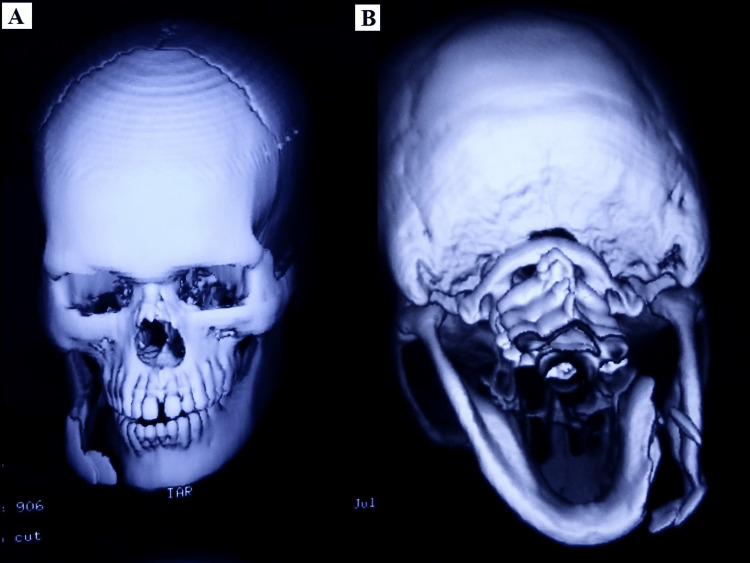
Pre-operative CT scan images showing comminuted body fracture of mandible. (A) Frontal view. (B) Posterior-anterior view.
